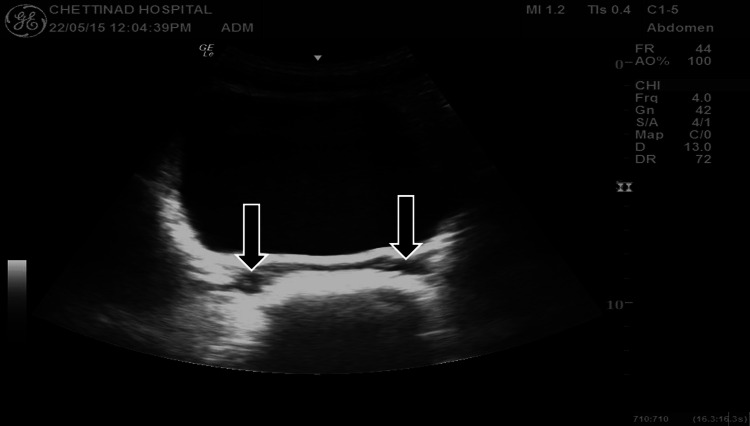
Ultrasound showing multiple tiny cystic lesions in bilateral seminal vesicles (arrows)
